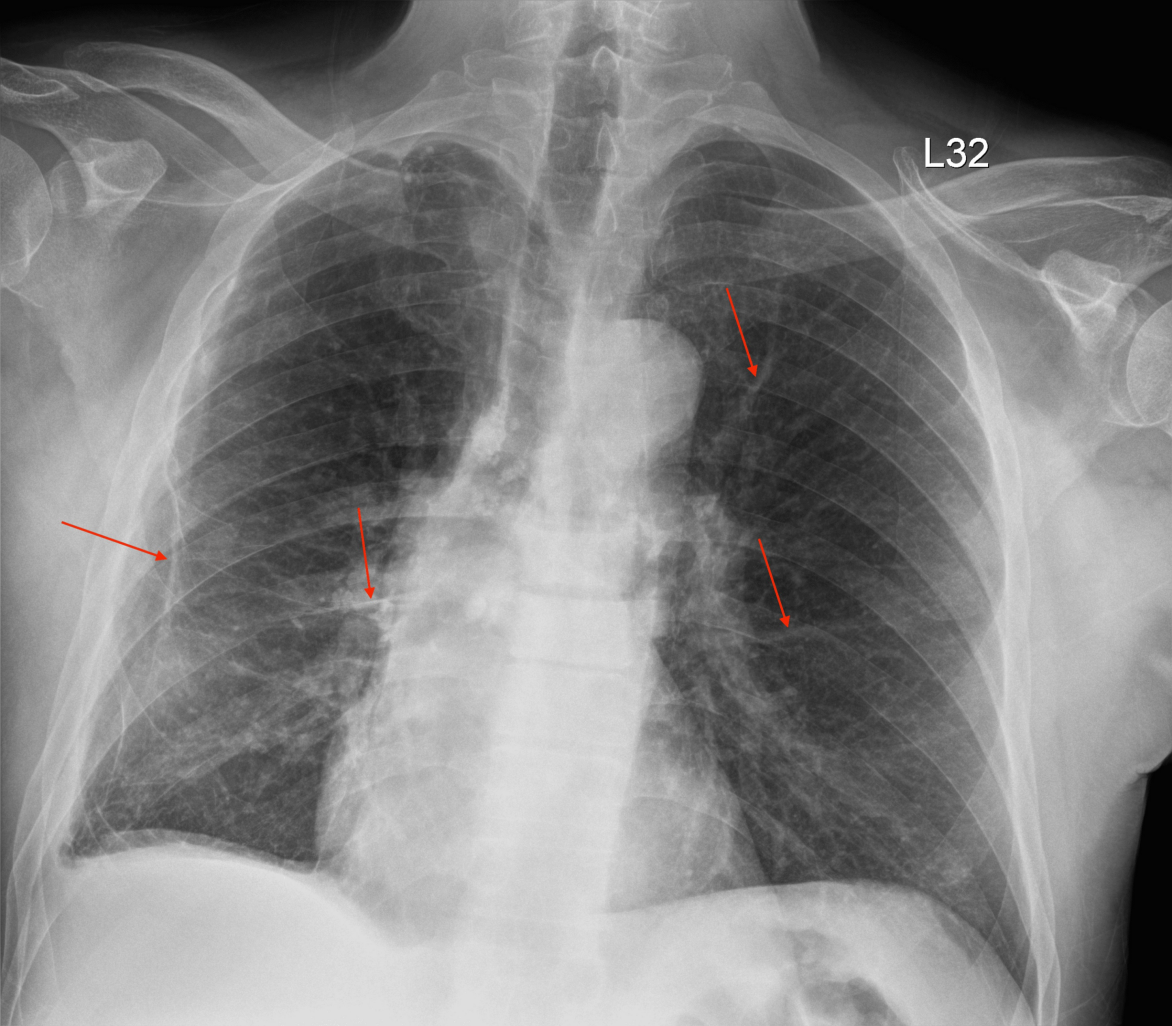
Preoperative chest X-ray Red arrows show known bullous emphysematous changes and scarring
